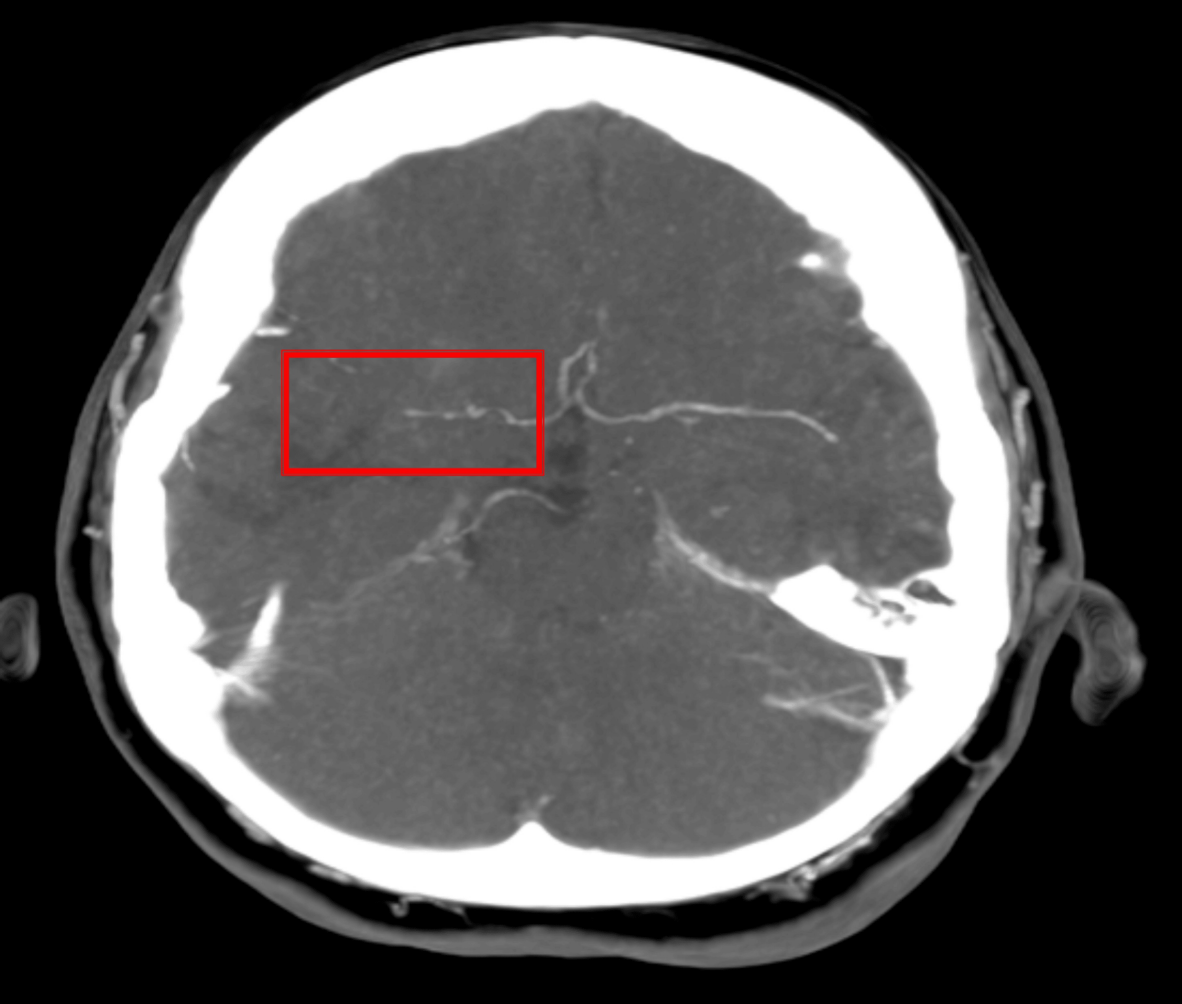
CT Angiogram showing segmental narrowing of the right MCA CT: computed tomography; MCA: middle cerebral artery
